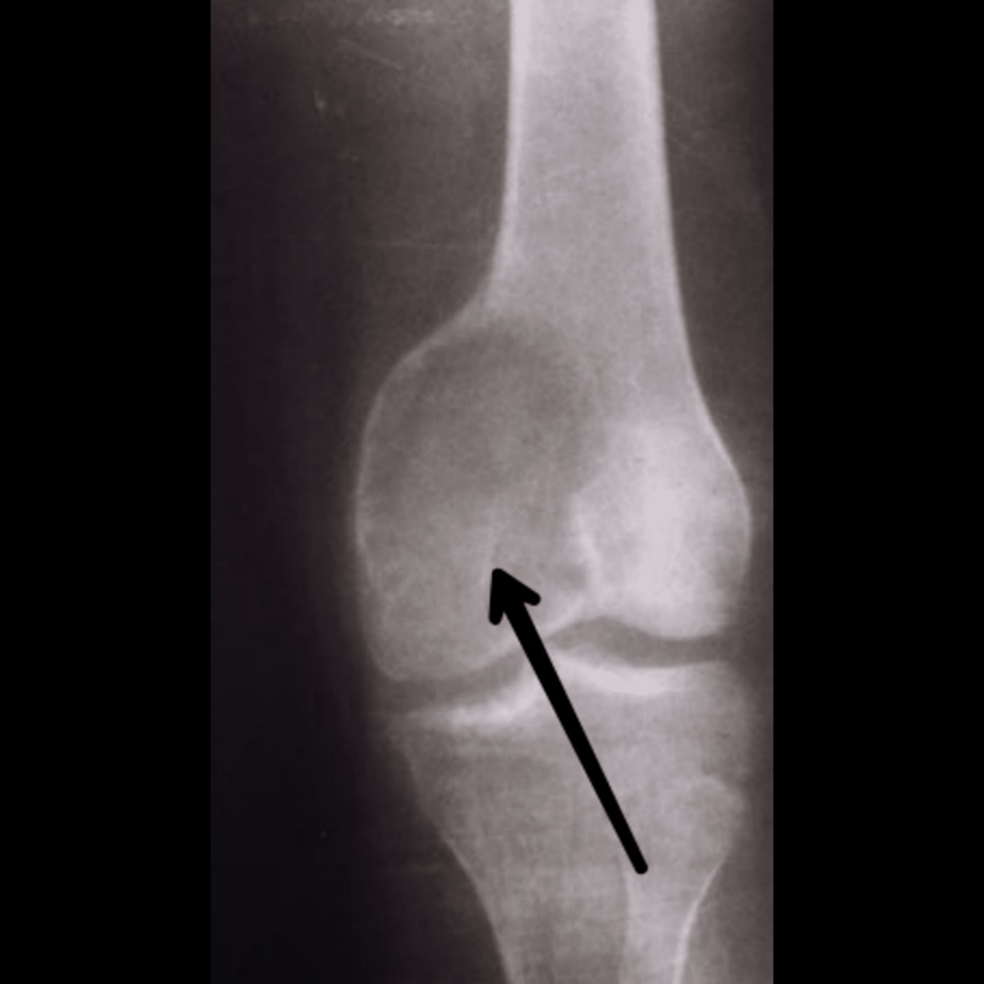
X-ray of knee joint showing GCTB in medial femoral condyle extending in the subarticular region. Image taken by Yash Jha. GCTB: giant cell tumour of bone
